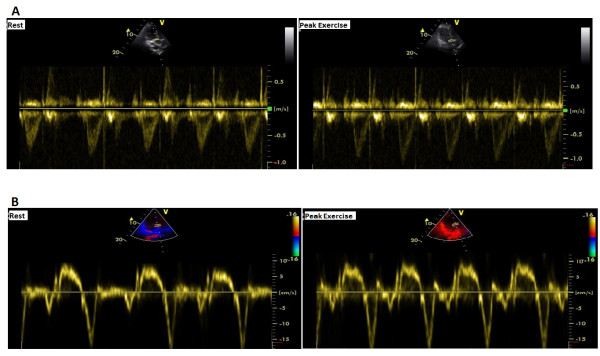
Exercise stress echocardiographic images. A: Measurements of left ventricular outflow tract velocities at rest and peak exercise, B: Basal septal tissue systolic velocities at rest and peak exercise, in a hypertensive 53-year-old woman with left ventricular hypertrophy [28].
